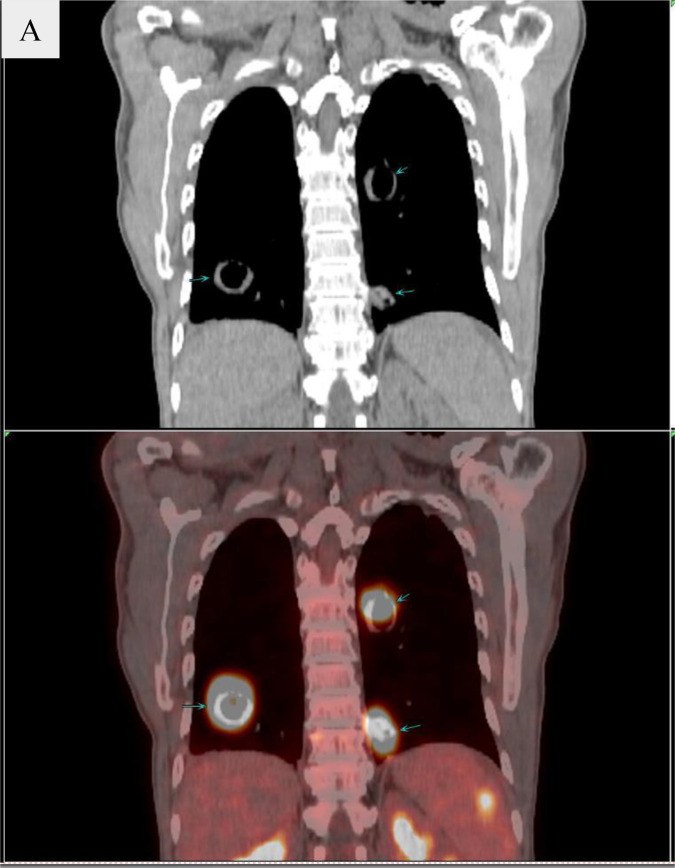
Images of PET‐CT. (A) shows High metabolism of the multiple thick-walled pulmonary cavities.
